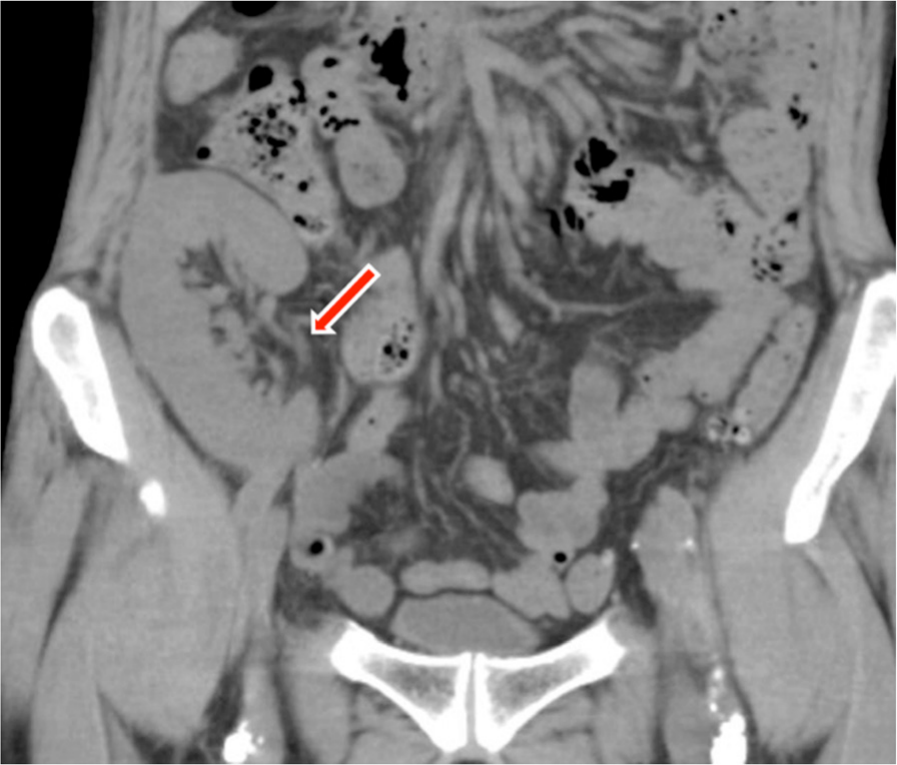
Non-enhanced abdominal CT scan (coronal) shows that the location of the transplanted ureter is unclear (*arrow*)
